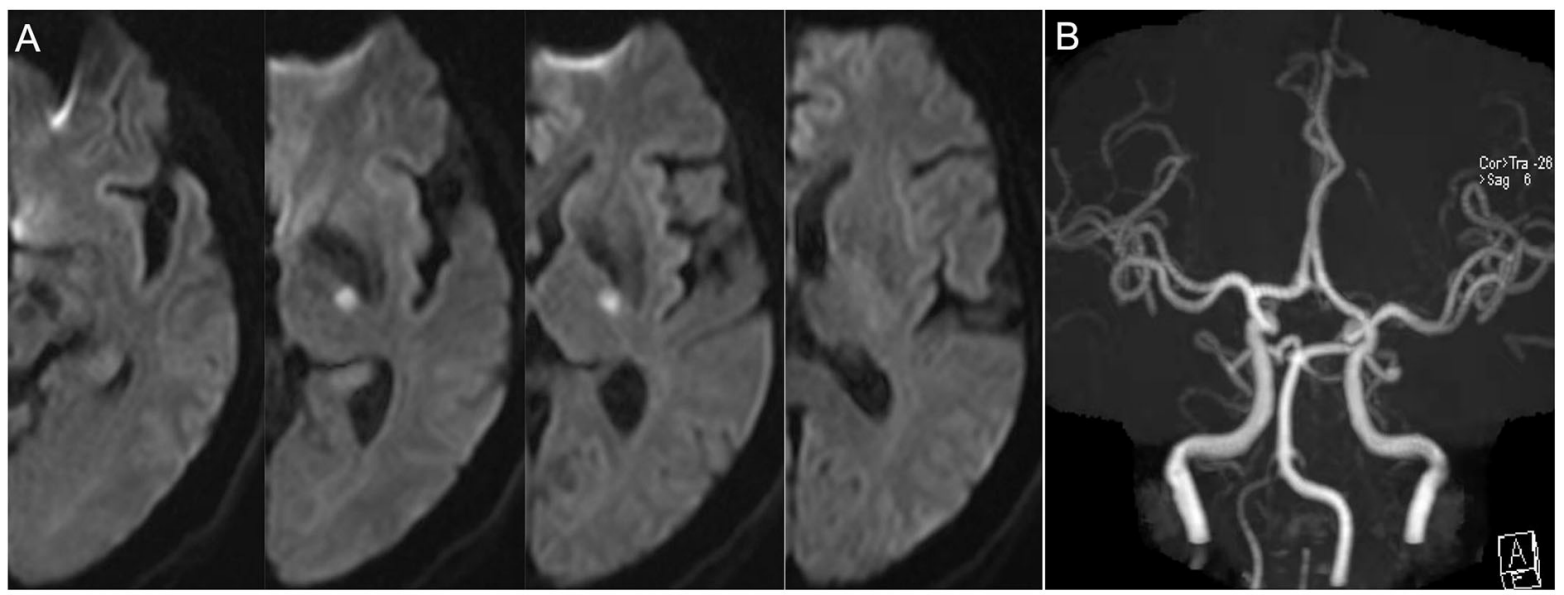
Representative case of non-progressive infarction. (A) The infarct slice number <3 on 5 mm thick axial DWI images and the patient had no progressive infarction. (B) MRA showed no obvious etiologies (vasculitis, dissection, Moyamoya disease) that led to ischemic stroke.
